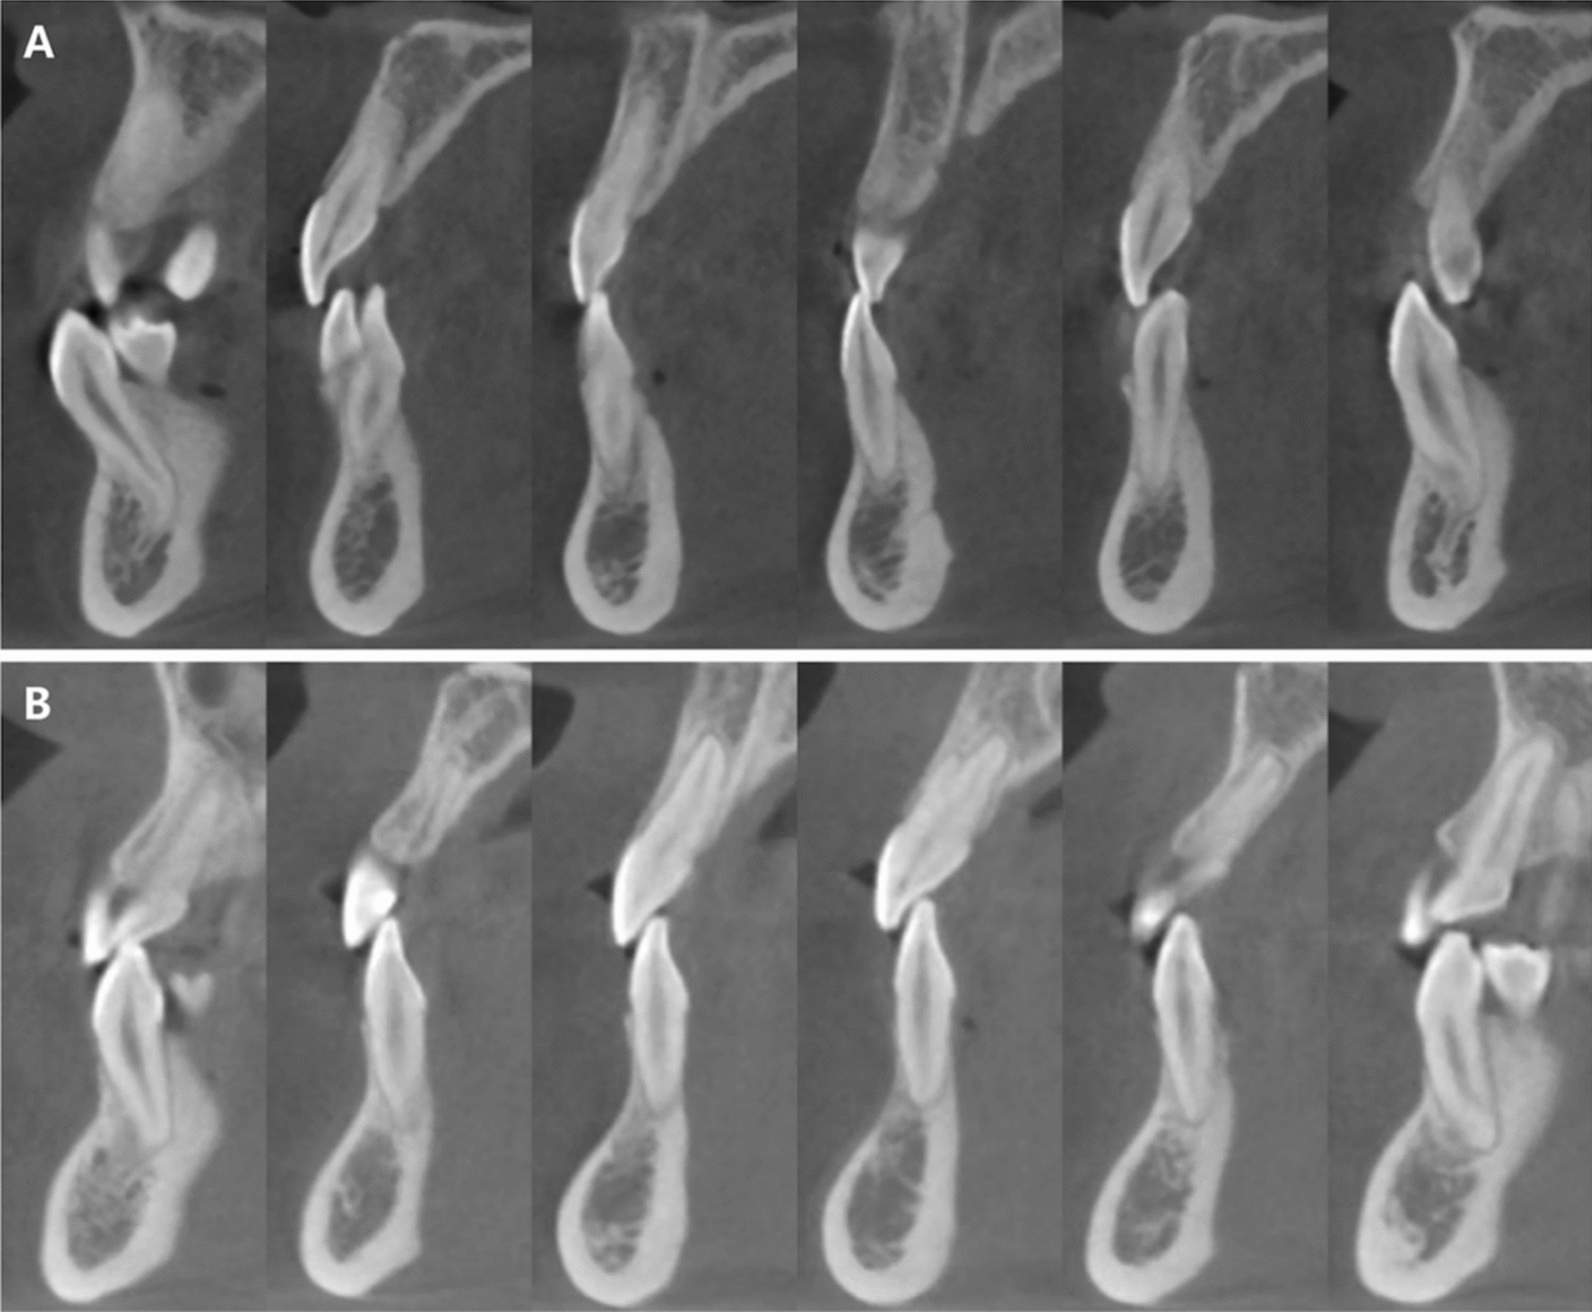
CBCT: Comparison incisors area at pretreatment (A) and posttreatment (B)
